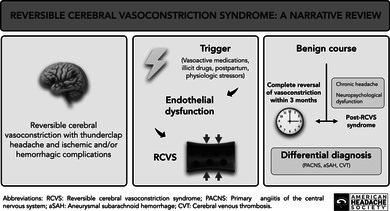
Abbreviations3D‐RAthree‐dimensional rotational angiographyA1first segment of anterior cerebral arteryA2second segment of anterior cerebral arteryaSAHaneurysmal subarachnoid hemorrhageASLarterial spin labelingBBBblood‐brain barrierCBFcerebral blood flowCGRPcalcitonin gene‐related peptidecSAHconvexity subarachnoid hemorrhageCSFcerebrospinal fluidCTAcomputed tomography angiographyCVTcerebral venous thrombosisDSAdigital subtraction angiographyFLAIRfluid‐attenuated inversion recoveryHELLPhemolysis, elevated liver enzymes, and low platelet countM1first segment of middle cerebral arteryM2second segment  of middle cerebral arteryMRAmagnetic resonance angiographymRSmodified Rankin ScaleP2second segment of posterior cerebral arteryPACNSprimary angiitis of the central nervous systemPIHpregnancy‐induced hypertensionPRESposterior reversible encephalopathy syndromeRCVSreversible cerebral vasoconstriction syndromeREVERCEreversible cerebral vasoconstriction syndrome international collaborative networkRNF213ring finger protein 213TCHsthunderclap headachesTGAtransient global amnesiaTKCtakotsubo cardiomyopathyVal66Metvaline‐66‐methionine substitution in the BDNF geneVWIvessel wall imagingWMHswhite matter hyperintensities
